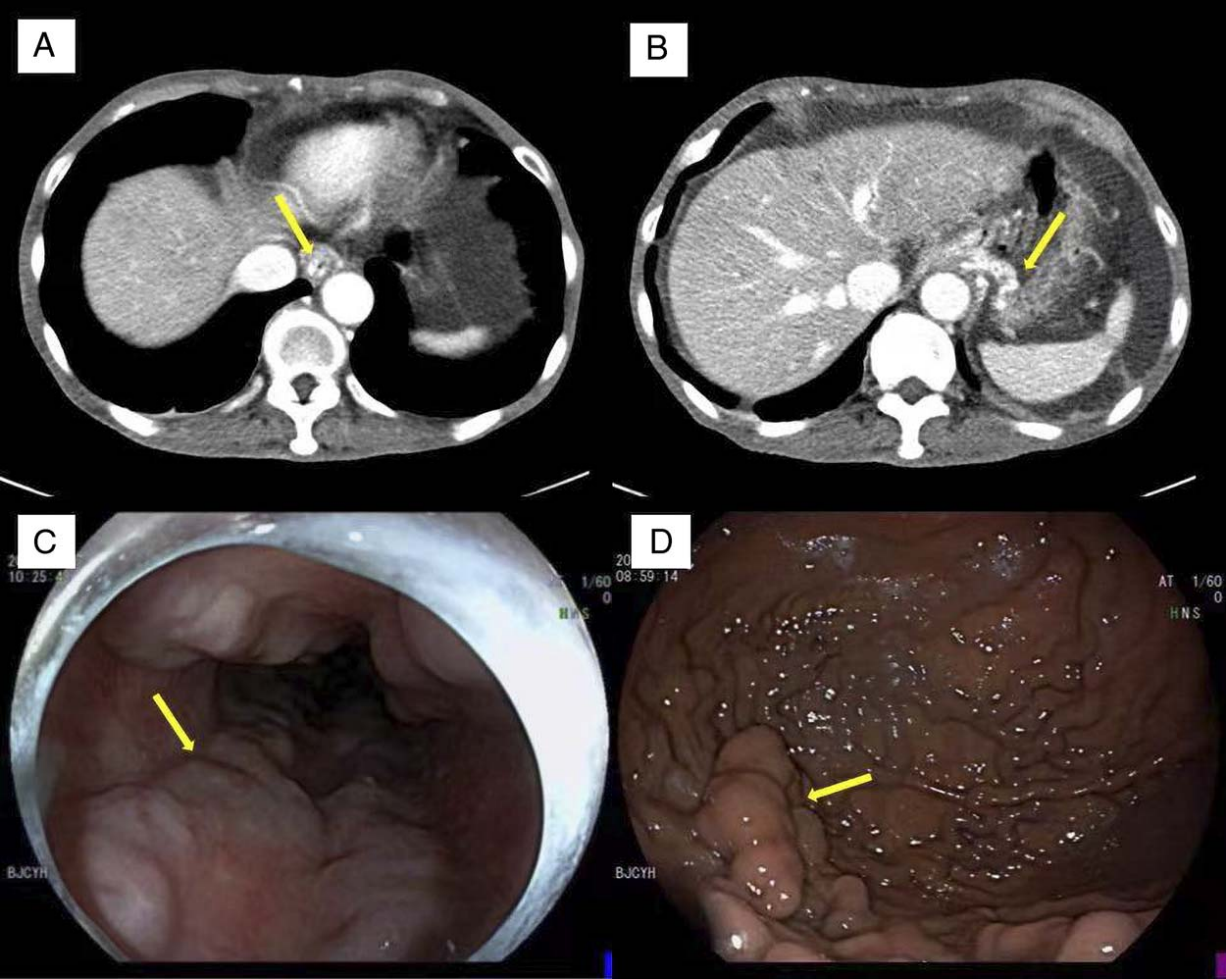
Varicose veins in patients at long-term follow-up. (A) Abdominal computed tomography (CT) showed a marked increase in the diameter of the lower esophageal vein. (B) Abdominal CT showed that the fundal veins were excessively tortuous and markedly thickened. (C) Gastroscopy showed four blue dilated blood vessels on the inner wall of the esophagus, and cherry red changes were visible on the surface of the vessels. (D) Gastroscopy shows nodular, beaded varicose veins in the stomach wall.
